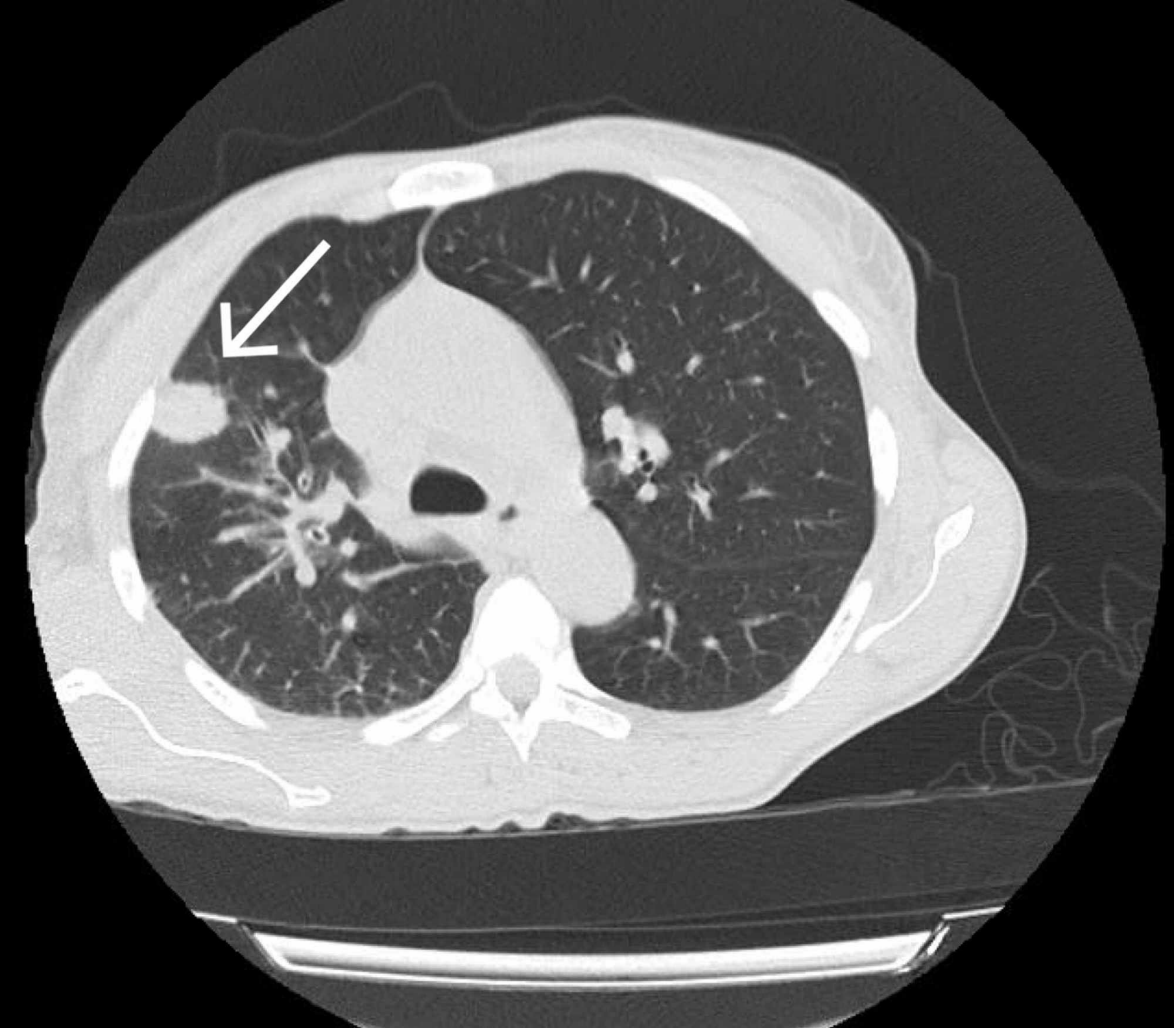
CT thorax demonstrating right upper lobe nodule measuring 1.9 x 1.6 cm CT, computed tomography
